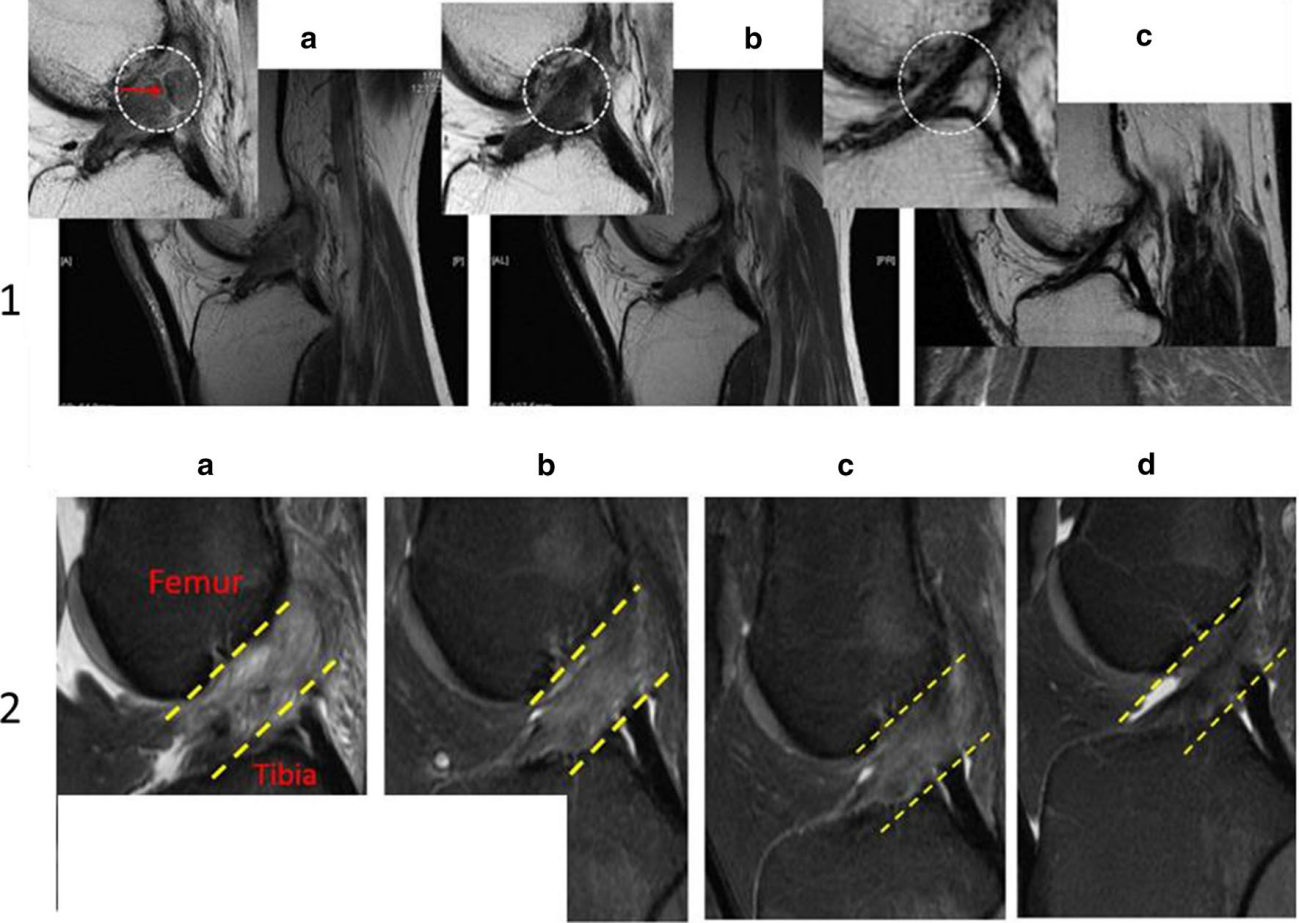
Progressions of ACL healing. 1 (a) MRI images of ACL for patient before receiving treatment. (b) ACL at 5 months post-treatment (medium–low signal intensity). (c) ACL at 9 months post-treatment (low signal intensity). 2 (a) MRI image of ACL pre-treatment. (b) ACL at 3 months post-treatment (medium signal intensity). (c) ACL at 6 months after stem cell procedure (medium–low signal intensity). (d) ACL at 11 months post-treatment (low signal intensity)
